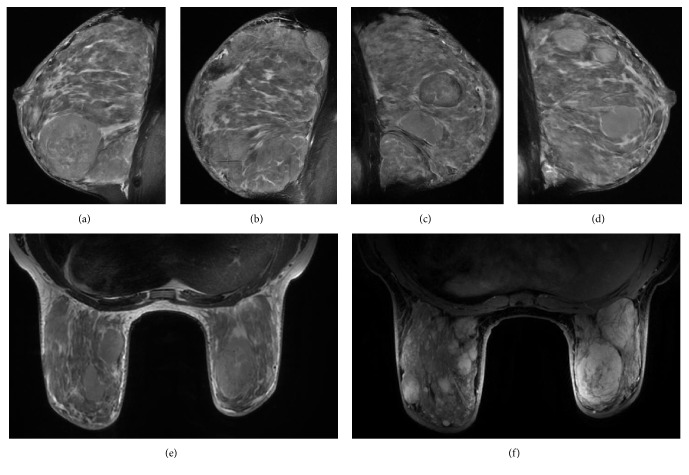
Magnetic resonance imaging findings. Right (a-b) and left (c-d) sagittal noncontrast fat suppressed T2-weighted image (T2W-FATSAT) showing extreme fibroglandular tissue and multifocal well-circumscribed masses, with internal linear reticular strands. Axial noncontrast fat suppressed T2-weighted image (T2W-FATSAT) (e) and axial contrast fat suppressed T1-weighted dynamic sequence after 6 minutes (T1WFATSAT+G) (f) demonstrating bilateral breast enlargement, with multiple circumscribed masses that present heterogeneous and persistent enhancement. Areas of nonmass enhancement with diffuse distribution also are observed in the inner and external quadrants of both breasts.
